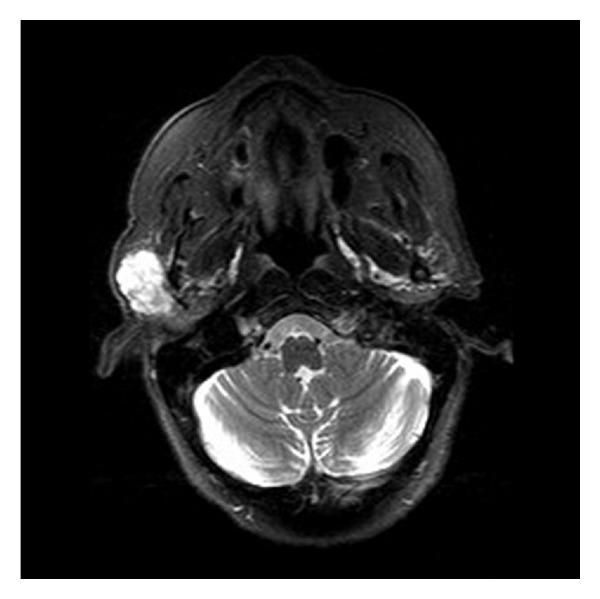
MRI demonstrating tumour in the right parotid gland.
